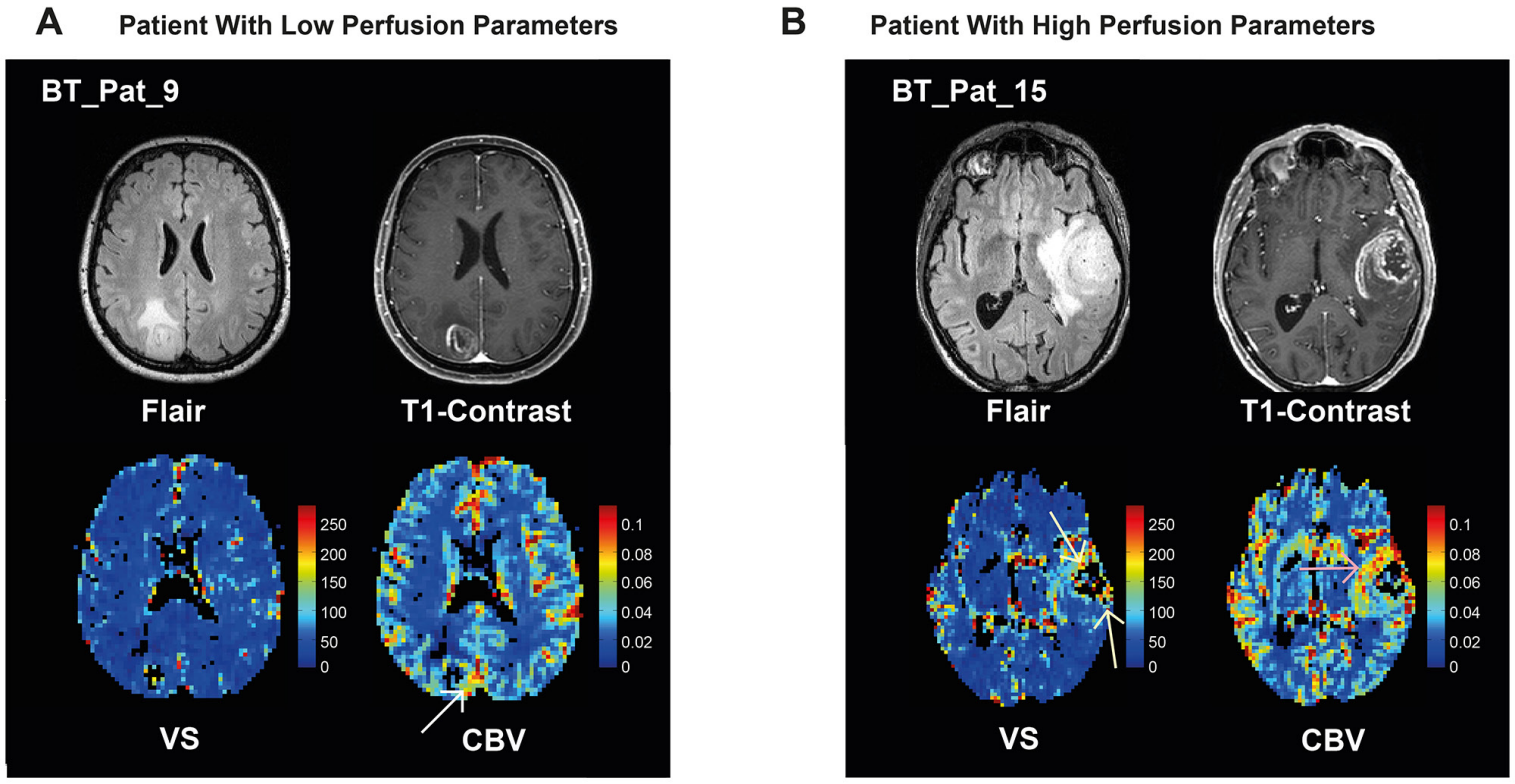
Examples of MR imaging A. 63 year-old female patient with a GBM in the right parietal lobe. A FLAIR hyperintense tumor with perifocal edema (top left) with rim-like contrast enhancement and a small central necrosis (top right) is present. The map of vessel size, VS, (bottom left) does not show any increased vessel size. On the CBV map (bottom right) only a shadowy increase of the CBV can be depicted at the rim of the tumor (whitw arrow). B. 76 year-old male patient with a GBM in the left temporal lobe. There is a FLAIR hyperintense tumor with perifocal edema (top left) with a thick area of contrast enhancement and a central necrosis (top right). The map of VS (bottom left) reveals increased vessel sizes on the anterior and posterior edges of the tumor (yellow arrows). On the CBV map (bottom right) a strong increase of CBV can be depicted predominantly at the anterior and medial rim of the tumor (red arrow). Both examples show that the VS and CBV calculations result in different non-concordant values in different tumor regions supporting the assumption that both parameters reflect different phenomena of tumor vascularization. The color-bars indicate CBV [ml/100ml tissue] or VS [μm].
